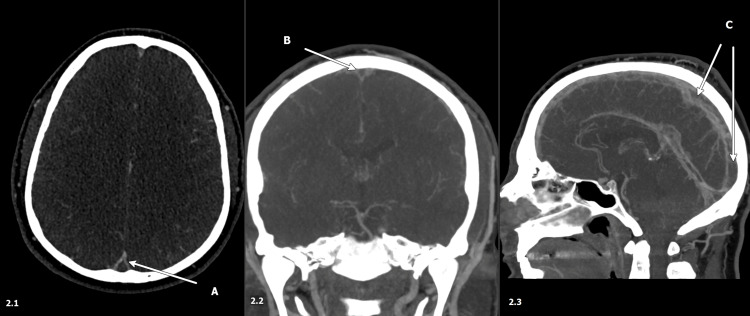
Superior sagittal sinus thrombosis on CTV. (A) Axial section demonstrating empty delta sign. (B) Coronal section demonstrating empty delta sign. (C) Sagittal section showing filling defect. CTV: Computed Tomography Venogram
